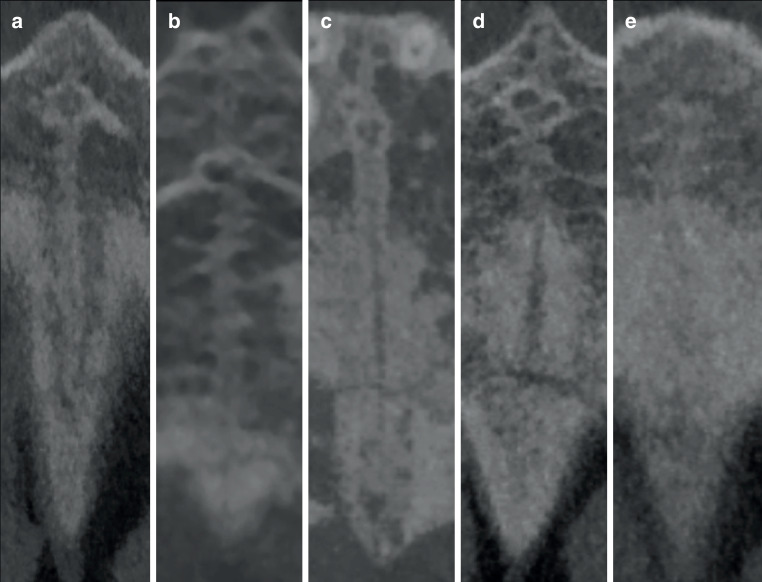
Cone-beam computed tomography (CBCT) images corresponding to the maturation stages by the Angelieri classification [8] of the midpalatal suture (MPS). a Stage A: almost straight high-density line; b stage B: scalloped high-density line; c stage C: two parallel, scalloped high-density lines; d stage D: fusion of the MPS only in the palatine bone; e stage E: fusion of the MPS in a portion of the maxilla Digitale Volumentomographie (DVT) entsprechend den Reifungsstadien nach der Angelieri-Klassifikation [8] der Sutura palatina mediana (MPS). a Stadium A: fast gerade Linie mit hoher Dichte; b Stadium B: gewellte Linie mit hoher Dichte; c Stadium C: 2 parallele, gewellte Linien mit hoher Dichte; d Stadium D: Fusion der MPS nur im Os palatinum; e Stadium E: Fusion der MPS in einem Teil des Oberkiefers
